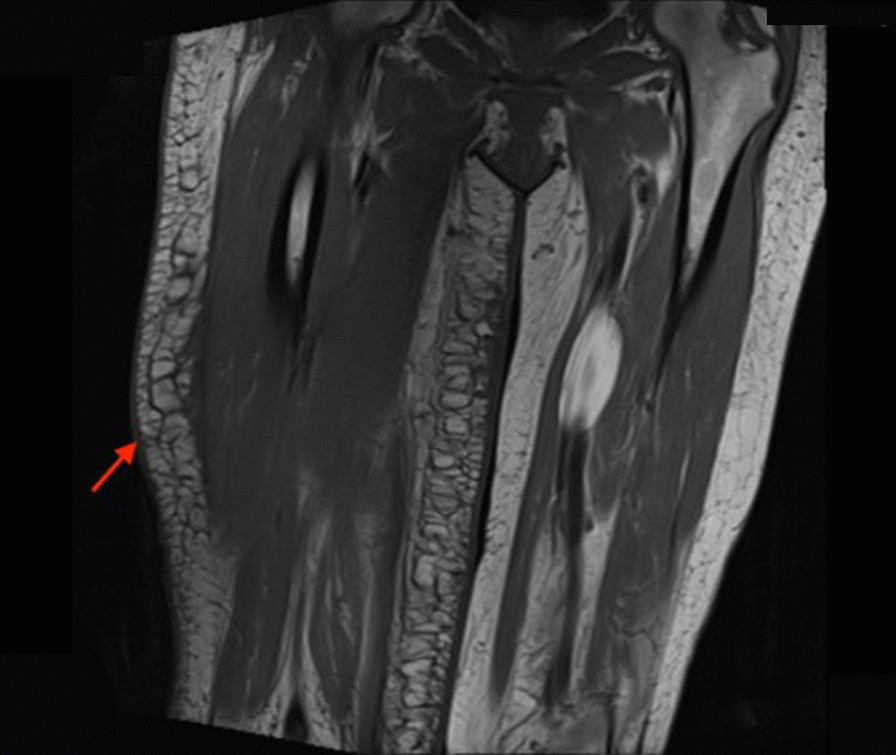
MRI right calf with arrow showing asymmetric increased edema within right thigh hypodermis
